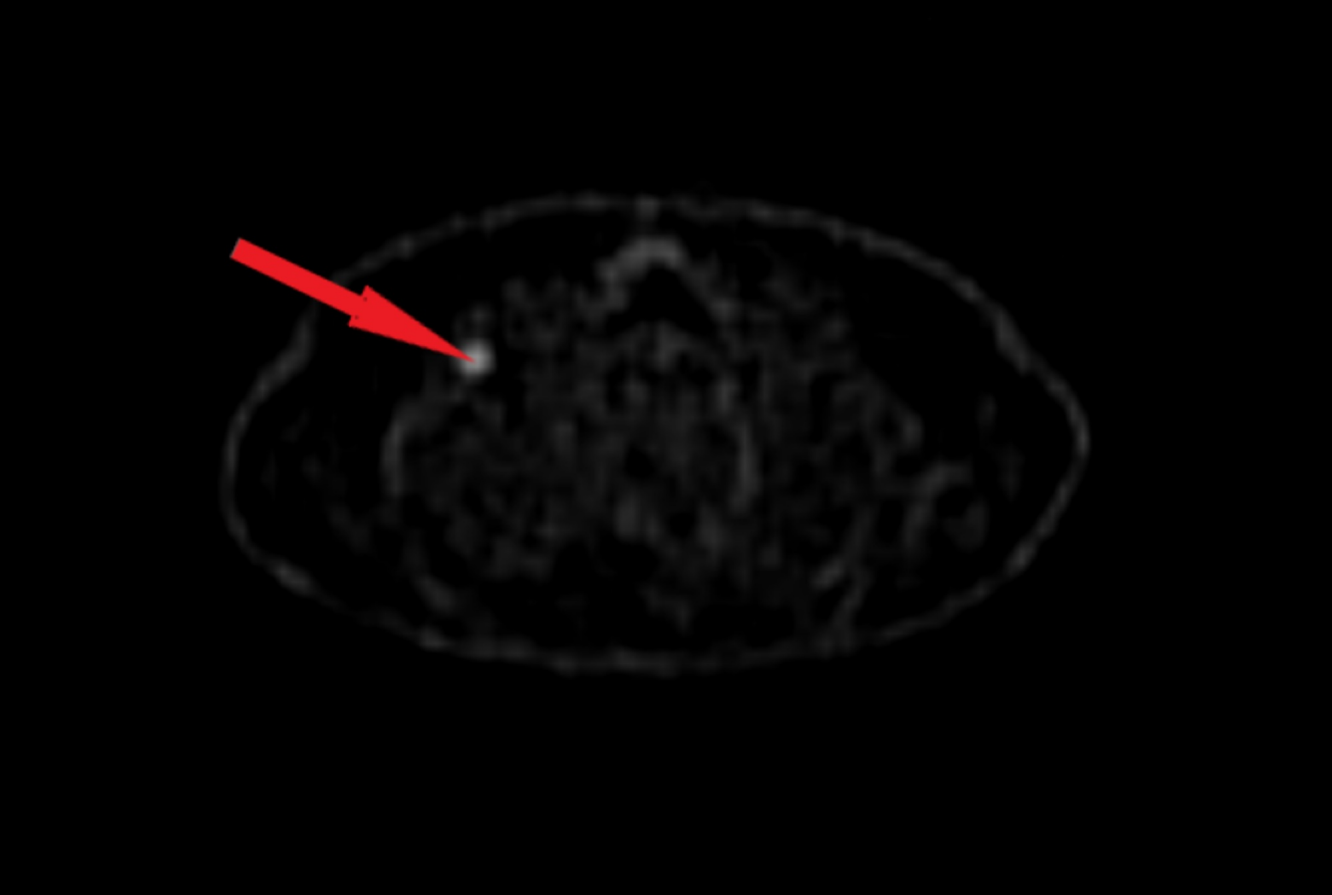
CT/PET scan A hypermetabolic nodule in the right lower lobe CT/PET: positron emission tomography-computed tomography
